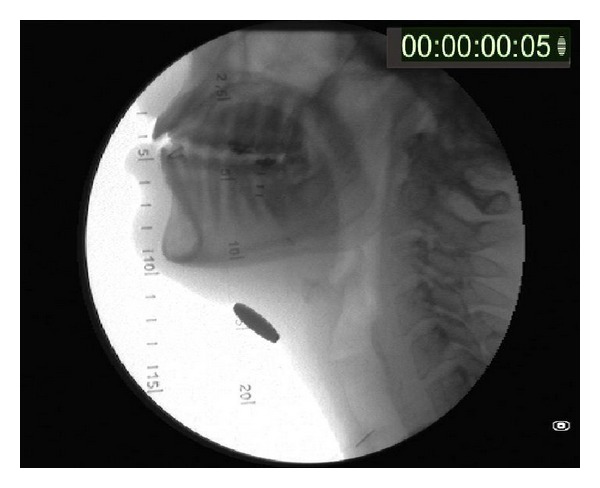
The anatomy of the upper aerodigestive tract is clearly identified in lateral cervical plain radiographs. Note the silhouette of the hard and soft palate, the tongue, including the tongue base, and the vallecula, and the epiglottis; the situation of the glottis can be easily estimated. The reference for the measurement of the distances is a 10 cents of eurocoin applied with adhesive tape to the midline of the neck (a constant size in any possible position: 20 mm), and there is a timer in the top right corner counting by the hundredth of a second.
